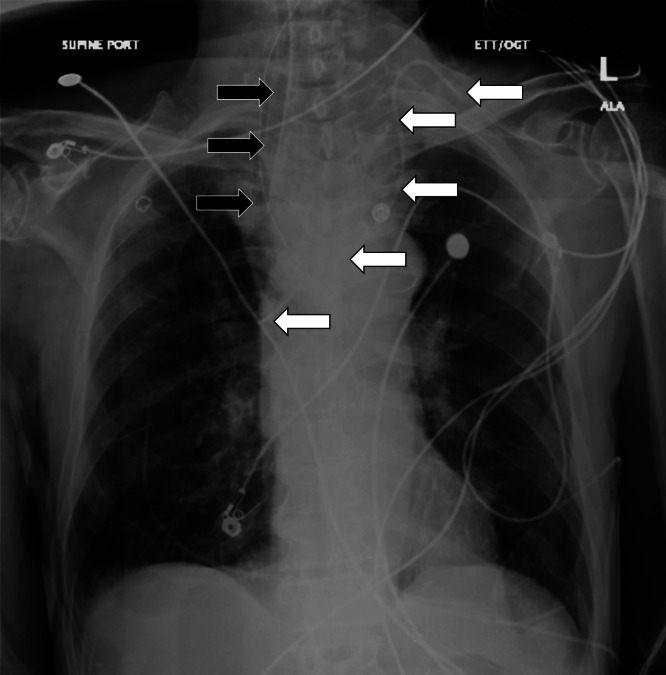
Repeat chest radiograph of a 77-year-old man after a rapid change in mental status showing the placement of an endotracheal tube (black arrows) and left internal jugular central line (white arrows).
